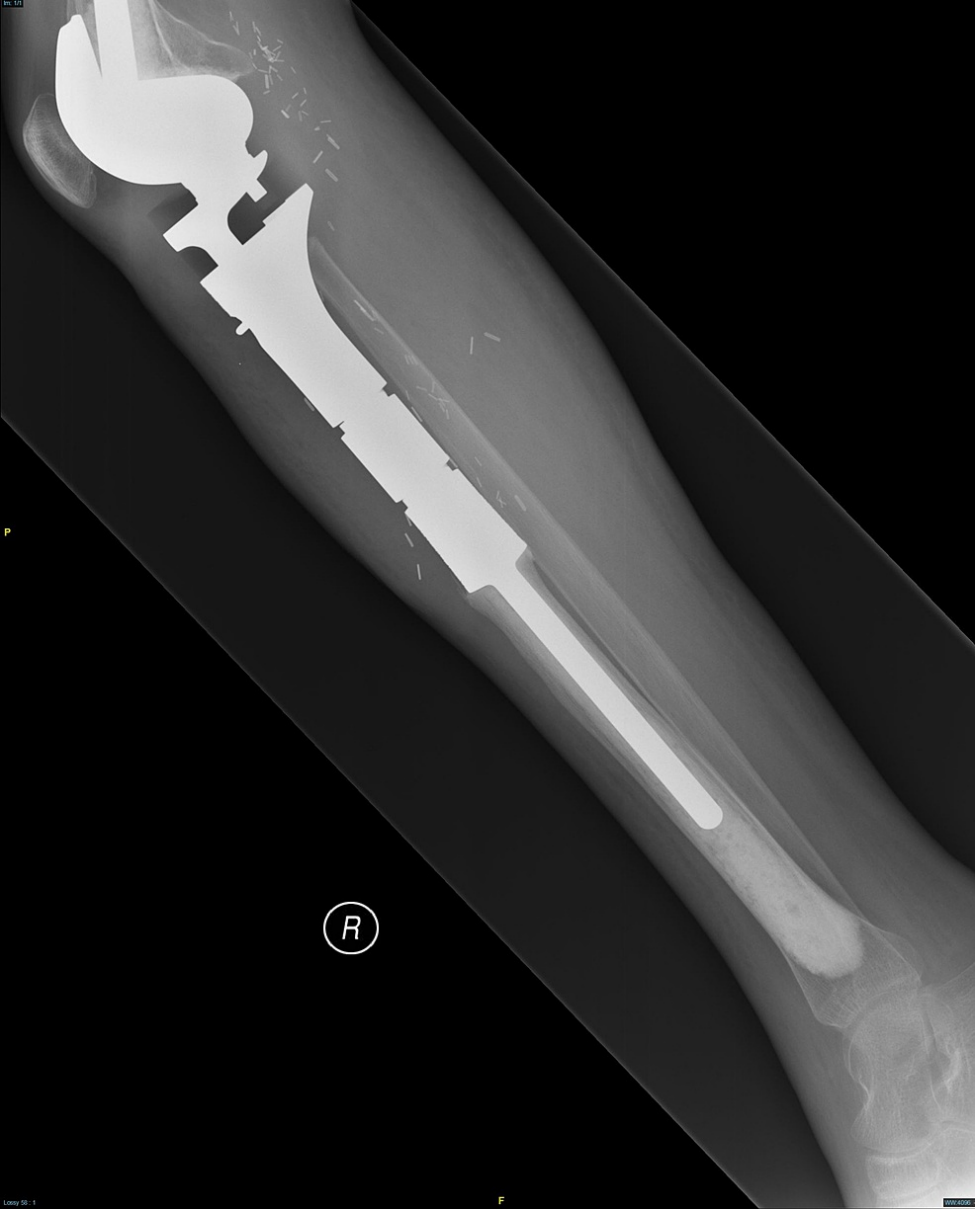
X-ray shows endoprosthesis in the right lower limb after resection of the tumor.
